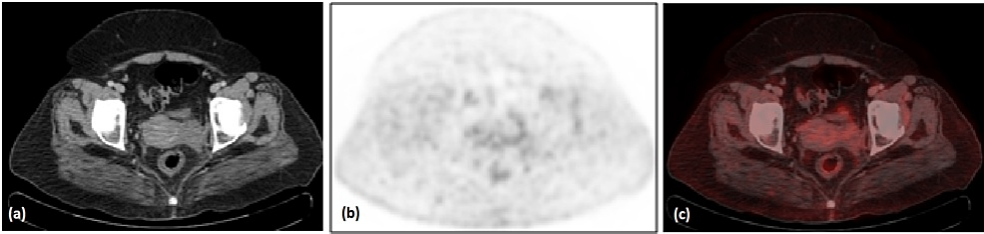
End of treatment pelvic CT (a), PET (b) and PET CT fusion (c) axial images showing complete metabolic response.
